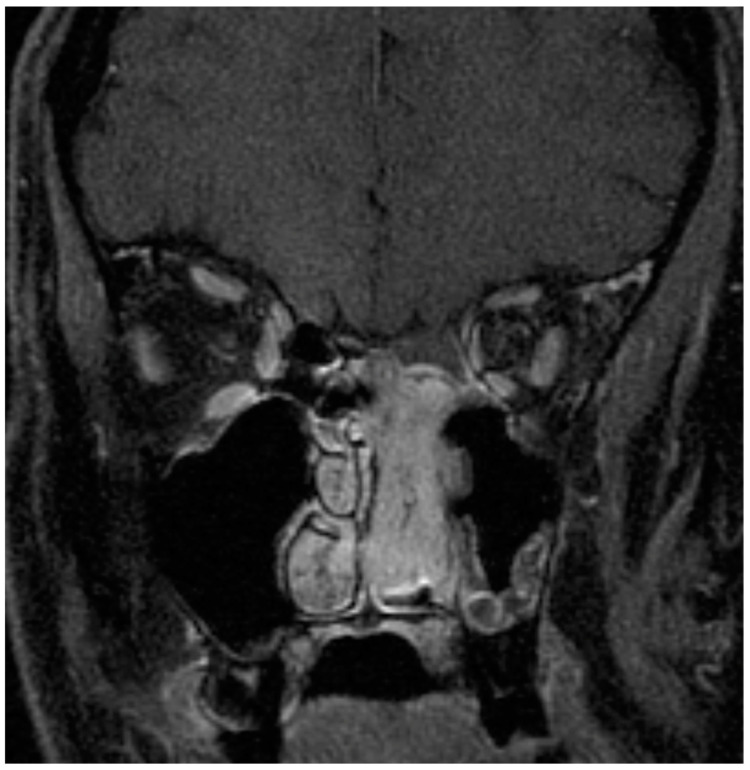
Magnetic resonance imaging for the diagnosis of olfactory neuroblastoma. A Kadish B tumor is seen here involving the nasal cavity and paranasal sinuses, not the orbits or intracranial cavity.
